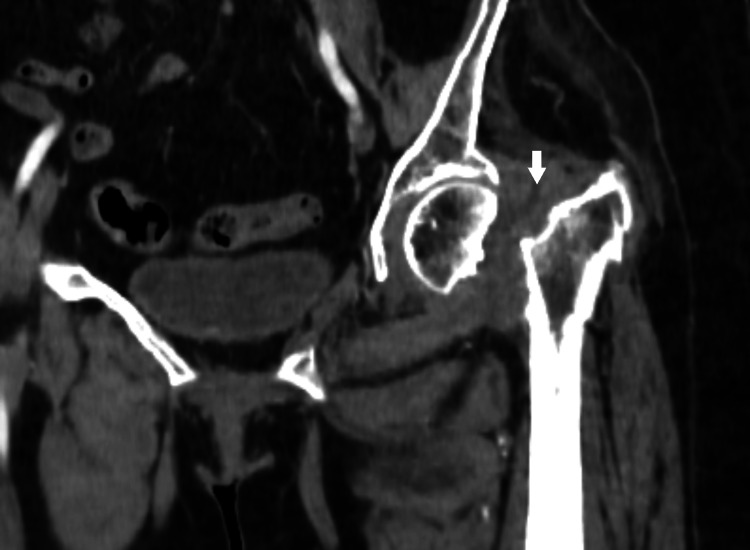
A non-contrast CT scan of the left hip in the front section showing the lesion
